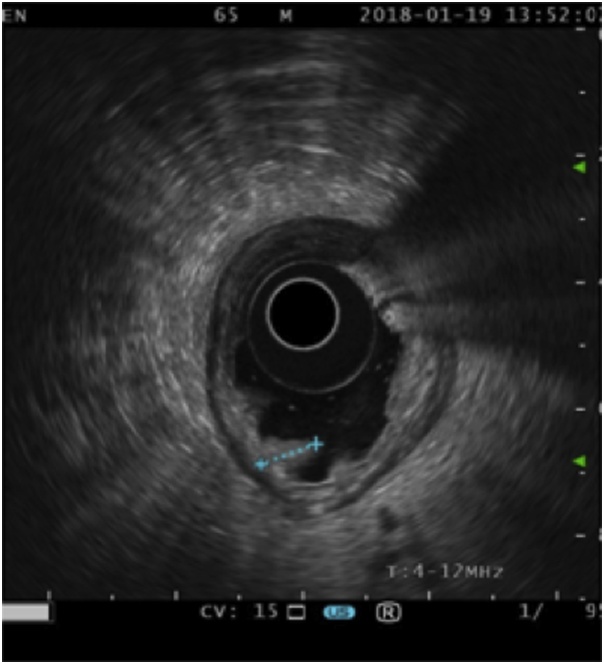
Endoscopic ultrasound showing a 32 mm adenocarcinoma (dots). The ulcerated tumor does not invade the muscularis.
